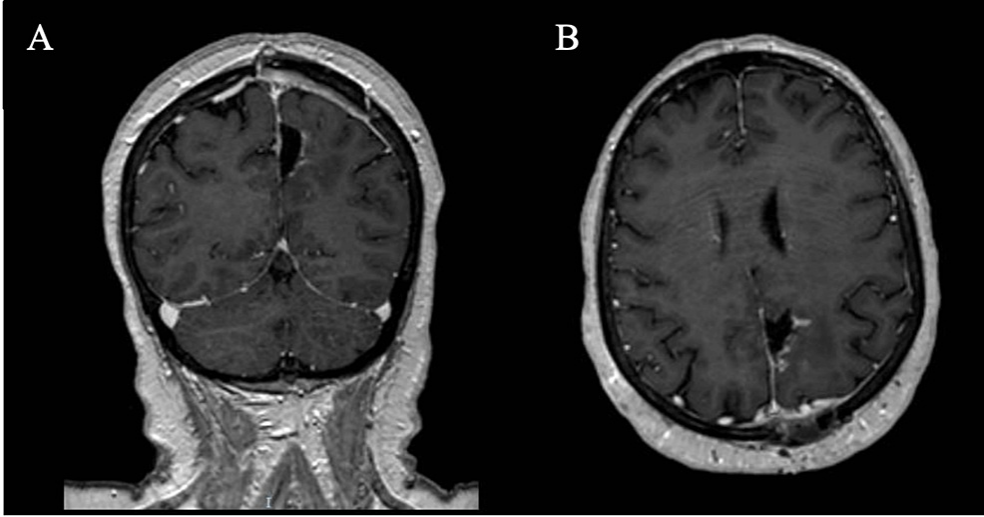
T1-contrasted MRI of the brain coronal view (A) and axial view (B) postoperatively, shows complete tumor resection.
